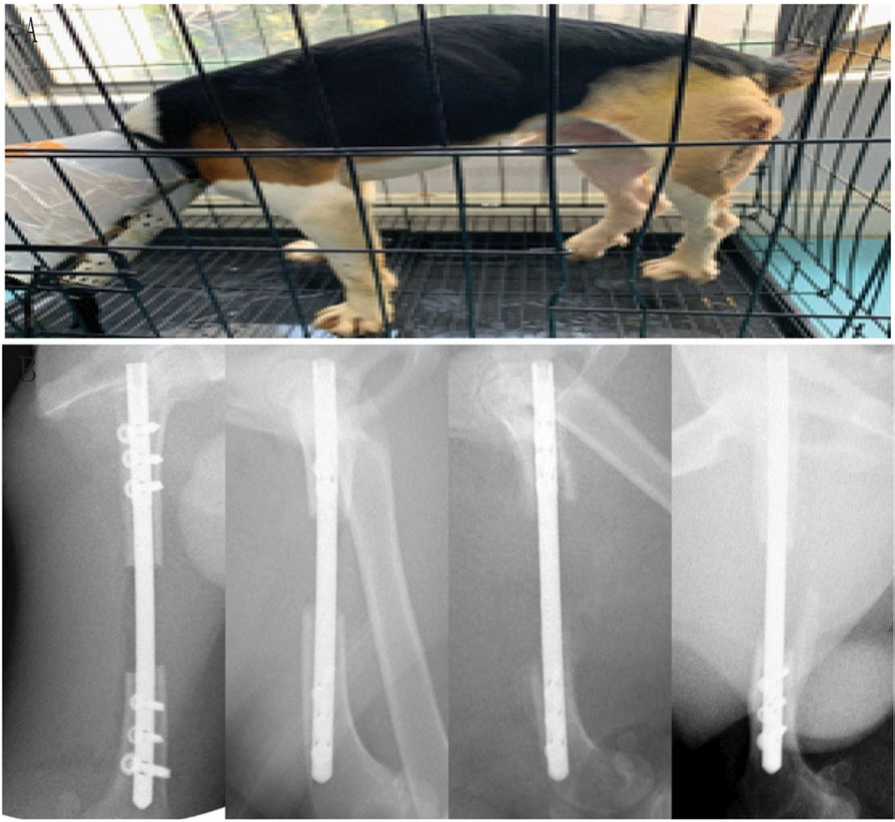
Postoperative conditions of the Beagle models showing: A Stable weight bearing on three limbs at 1 week; B X-ray radiographs postoperatively, and at 1, 2 and 3 months follow-up
